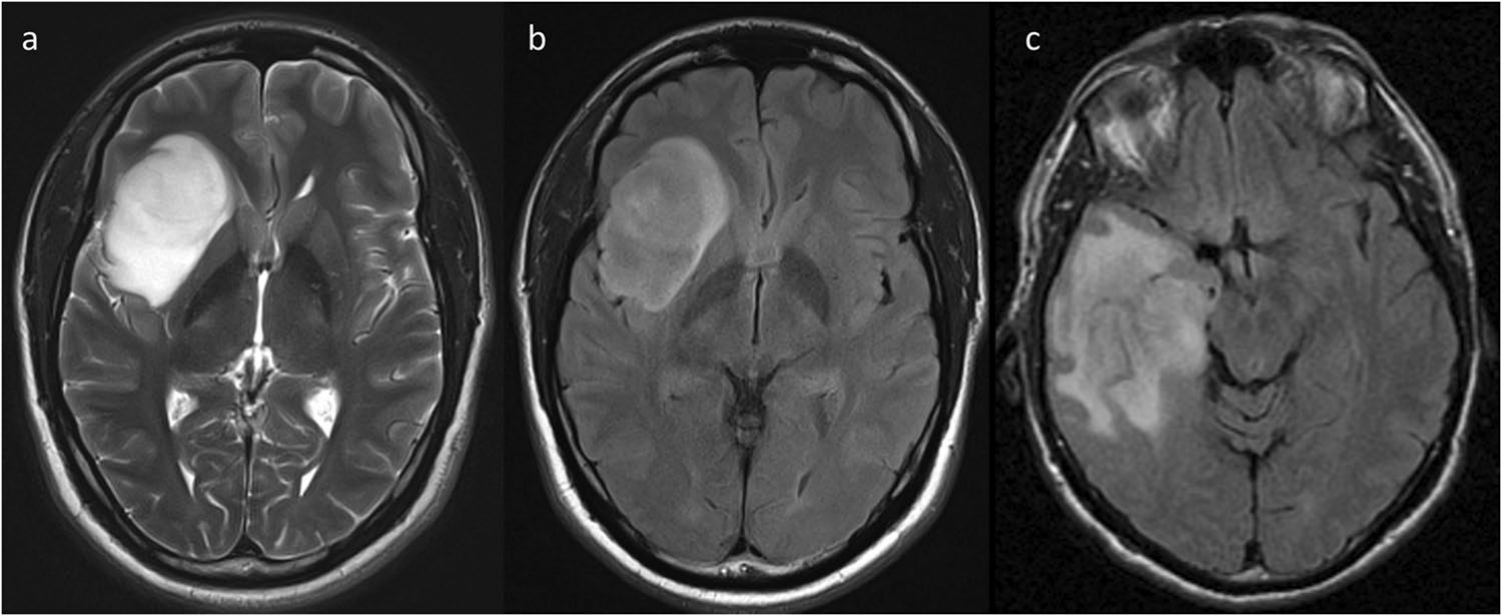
Representative patient images of the T2-FLAIR mismatch sign and tumour margins. The well-defined right fronto-insular tumour (a and b) demonstrates high T2 signal on T2-weighted imaging (left), with the majority of the lesion demonstrating substantially lower signal on FLAIR (middle), with a preserved FLAIR-hyperintense rim. In contrast, the right temporal tumour (c) in a different patient has ill-defined margins
